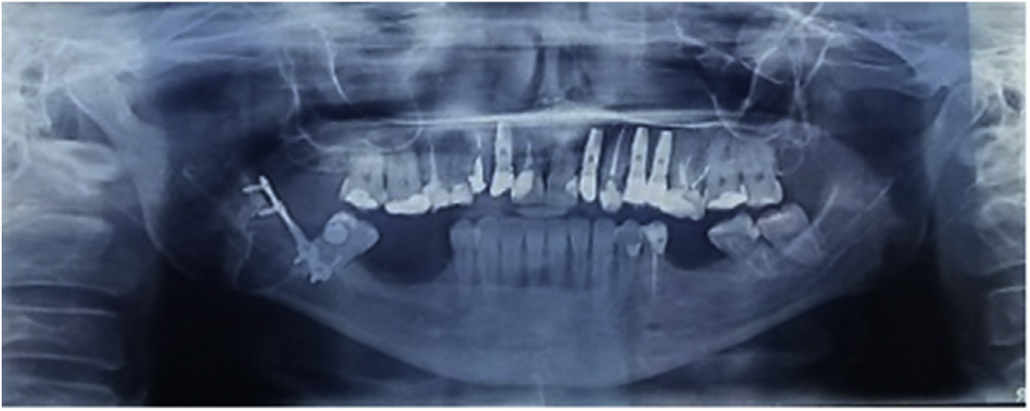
Post operative Radiography (7 days).
